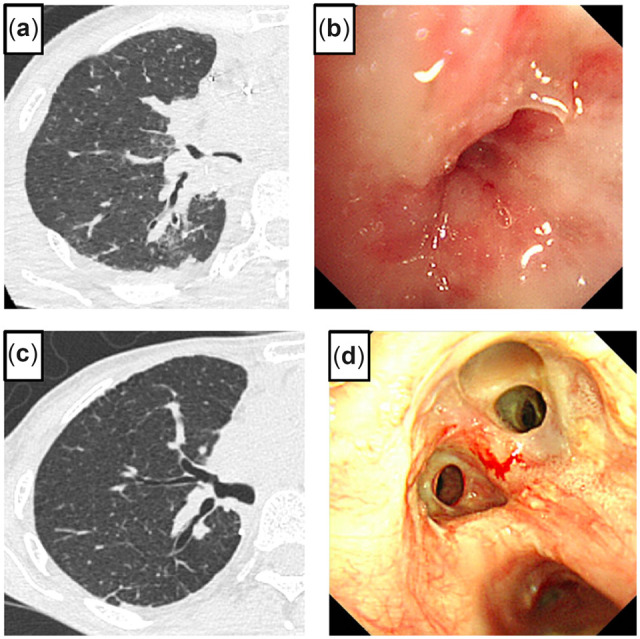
Chest computed tomography shows kinking of the anastomosed right upper bronchus (RUB) (a). Bronchoscopy before rethoracotomy shows narrowing of the anastomosed RUB (b). A chest computed tomography scan shows passage of the re-anastomosed RUB (c). Bronchoscopy shows healing and passage of the re-anastomosed RUB (d).
